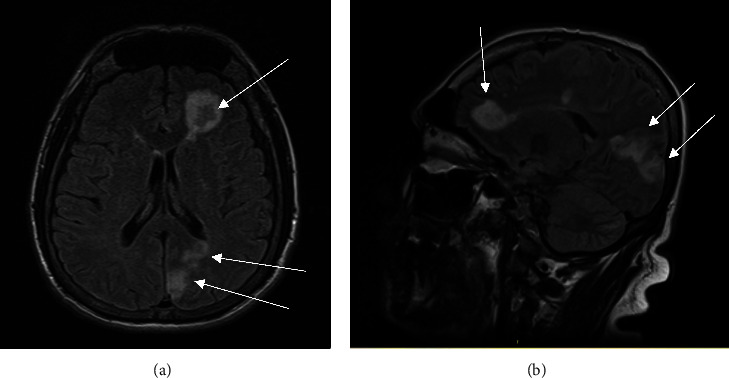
(a) Axial and (b) sagittal views of initial MRI imaging displayed left frontal, occipital, and parietal lobe abscesses with leptomeningeal enhancement.
